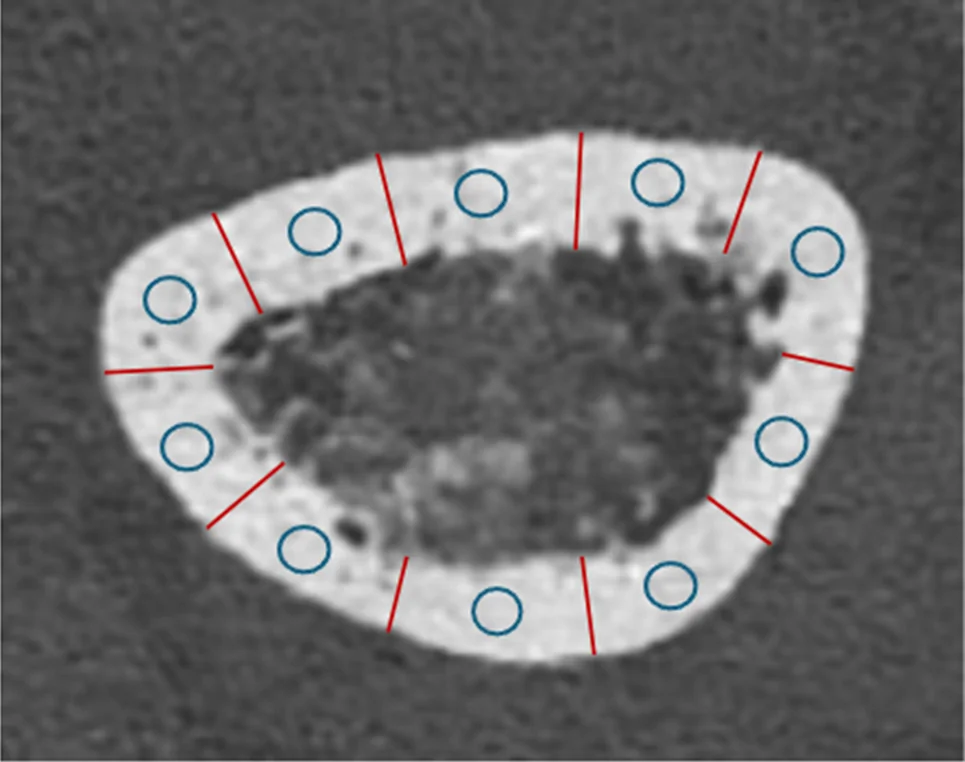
Axial image of distal radius with red lines representing measurements of cortical thickness, and blue circles denoting regions of interest (ROI) for calculation of average CT number (HU) in cortical bone
